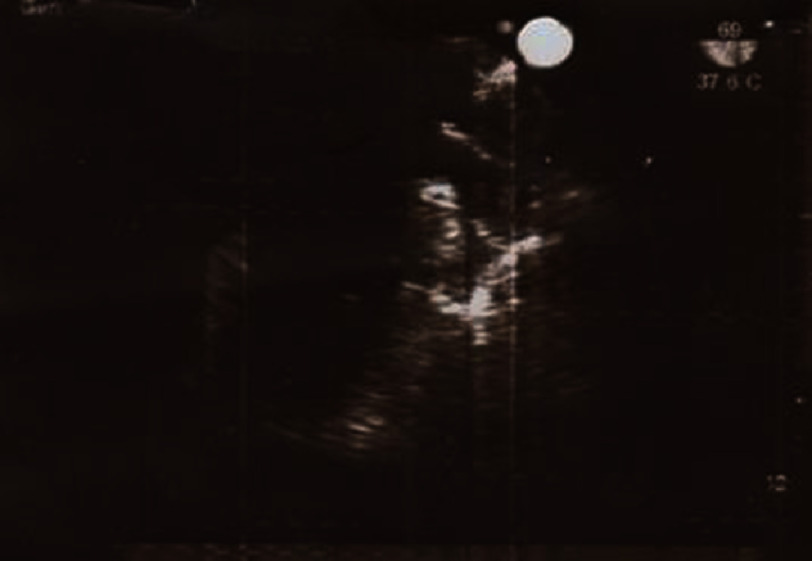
Transesophageal echocardiography from a different angle showing thrombosis in opening of inferior vena cava.
